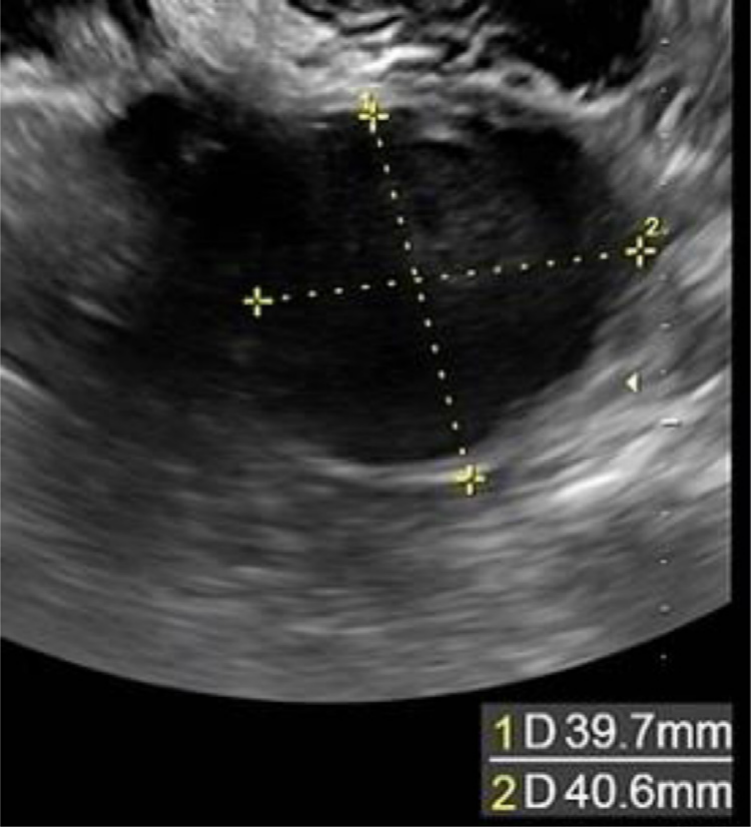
Ultrasound imaging before pregnancy. A 40-mm ovarian endometriotic cyst was noted.
